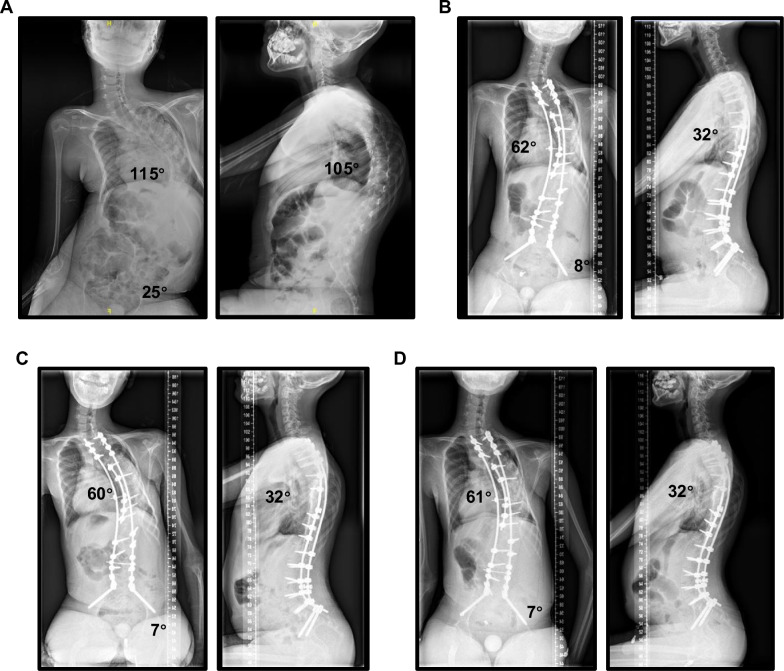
A 12-year-old male diagnosed with SMA underwent posterior spinal fusion and pelvic fixation by using S2-AI screws (both side: 8.0 * 80 mm). (A) Preoperative posteroanterior and lateral radiographs of the sitting whole-spine showing severe scoliosis (main curve cobb angle: 115°) with pelvic obliquity (25°). (B) shows that spinal deformity and pelvic obliquity were corrected at 6-mo postoperatively. (C) and (D) show that the corrected spinal deformity and pelvic obliquity were maintained at 18-months and 42-months postoperatively, respectively
